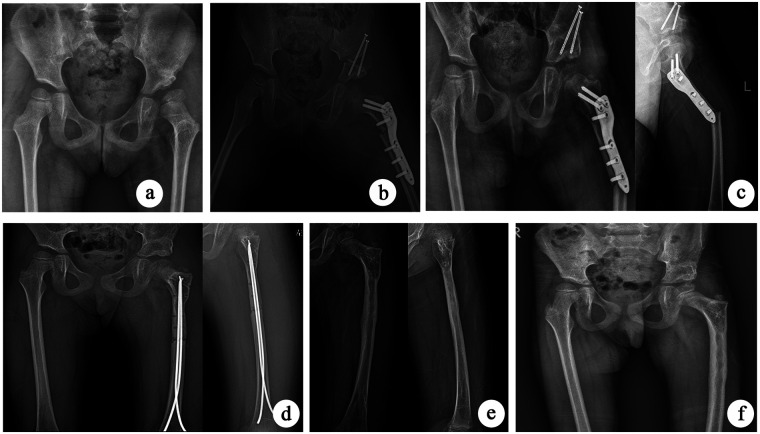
Left DDH in a 5-year-old girl. (a) Preoperative radiograph. (b) After Salter and proximal femoral osteotomy. (c) She had a femoral fracture in the screw hole at 9 months postoperatively when her femur was injured after a domestic fall. (d) ESIN was performed after removal of internal fixation. (e) Six-month radiograph after ESIN was removed. (f) Twelve-month radiograph after ESIN was removed. DDH, developmental dysplasia of the hip; ESIN, elastic stable intramedullary nailing.
